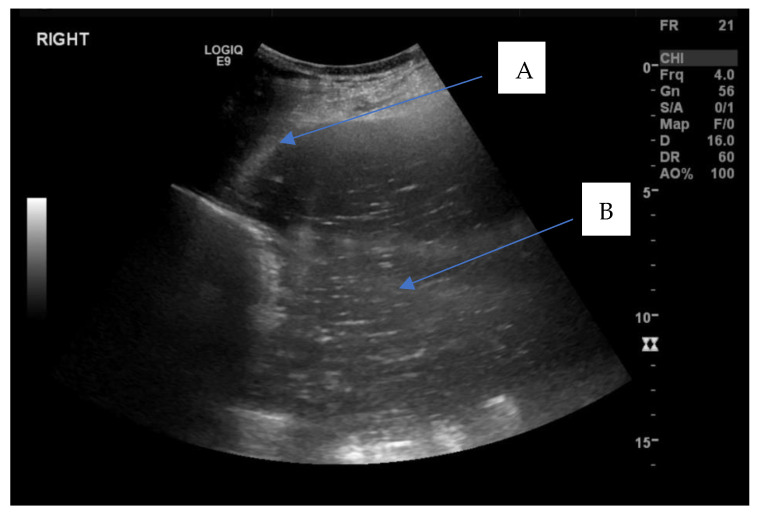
Transabdominal ultrasound showing a distended uterus filled with hyperechoic fluid within uterine lumen. A—uterine wall, B—large volume (>10 cm depth) of mucopurulent intrauterine fluid.
